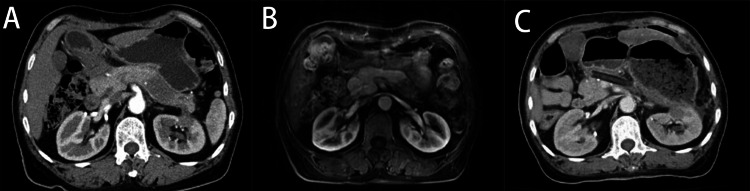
Abdominal computed tomography scan (A) and Magnetic resonance imaging (B) demonstrate a tumor in the tail of pancreas. Abdominal computed tomography (C) showed intra-abdominal free gas, peri-gastric exudation and possible discontinuity of gastric wall.
